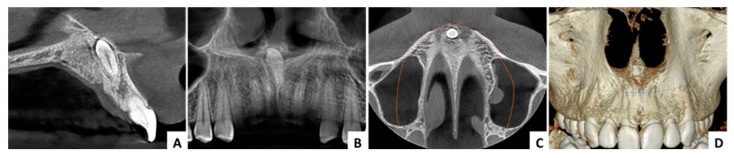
Case 3—radiological aspects: (A) CBCT cross-sectional view, (B) CBCT panorama view, (C) CBCT axial view, (D) CBCT 3D reconstruction.
